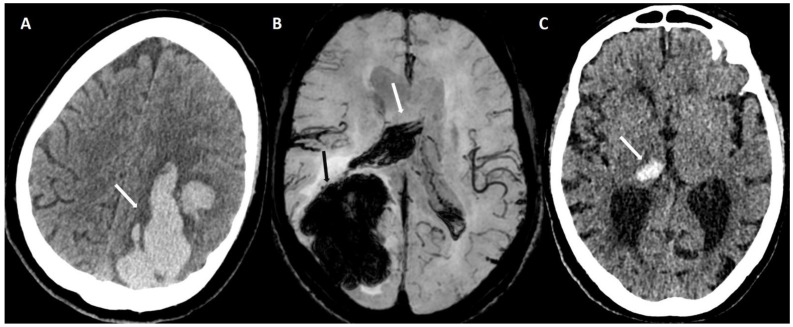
(A) Left parietal lobar hemorrhage (white arrow) on non-enhanced CT (100 kV, 596 mAs, slice thickness 1 mm), (B) right occipital lobar hemorrhage (black arrow) with intraventricular extension (white arrow) on axial venBOLD MRI (1.5 T, TE = 49 ms, TR = 34 ms, 10 mm MinIP). (C) Deep-seated right sided thalamic hemorrhage (white arrow) on non-enhanced CT (100 kV, 394 mAs, slice thickness 1 mm).
